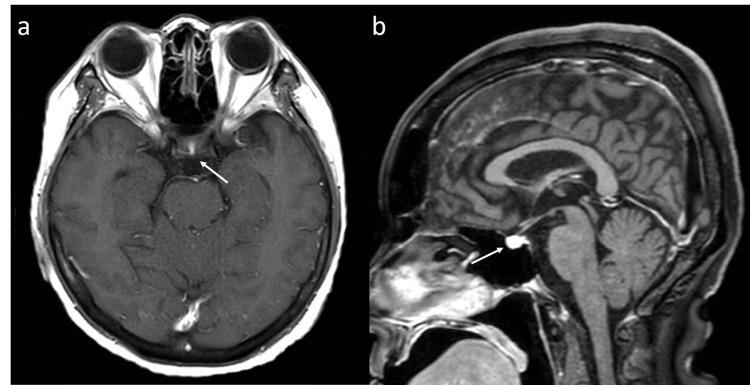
Magnetic resonance imaging of the head revealed no obvious abnormal findings in the pituitary gland (arrows) (a, sagittal plane; b, horizontal plane). Intracranial metastatic lesions were not observed.
